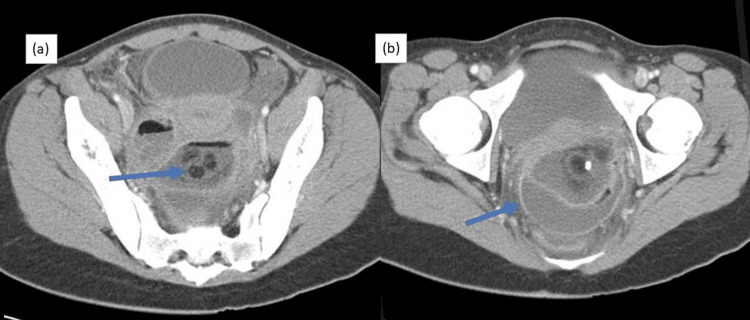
CT of the abdomen and pelvis showing the presence of three heterogenous rounded masses (a) and smooth enhancement of the peritoneal wall with free fluid in the abdominal cavity (b). CT, computed tomography
